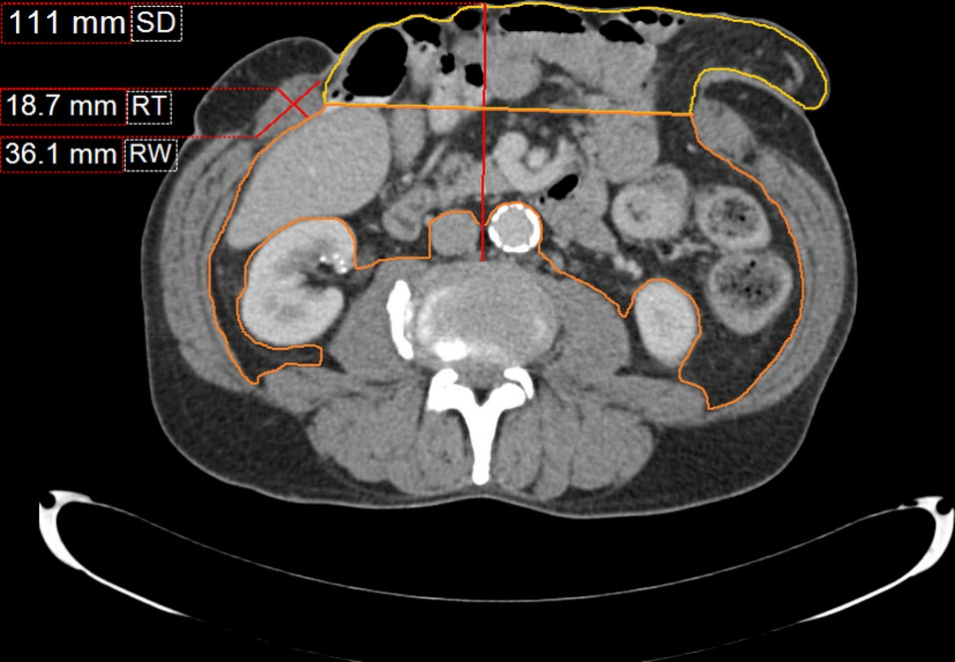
CT measurements. The orange outline represents the intra-abdominal area whereas the yellow outline represents the hernia sac. SD, RT and RW can be seen on the left. (Color figure online)
